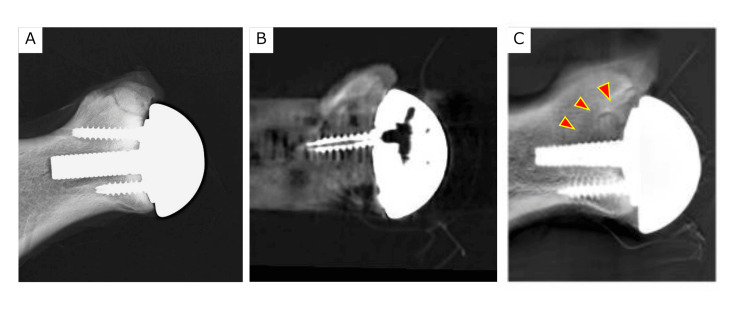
Anterior glenoid bone defect model a. radiographic evaluation, b. coronal CT image, and c. tomosynthesis. Arrowheads show areas of the anterior glenoid bone defect.
